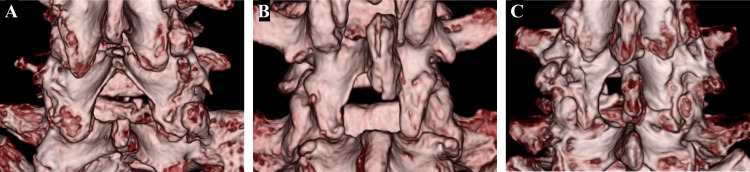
Three-dimensional computed tomography images after surgery. Three-dimensional computed tomography images after bilateral partial laminectomy (A), lumbar spinous process-splitting laminectomy (B), and microendoscopic laminotomy (C).
